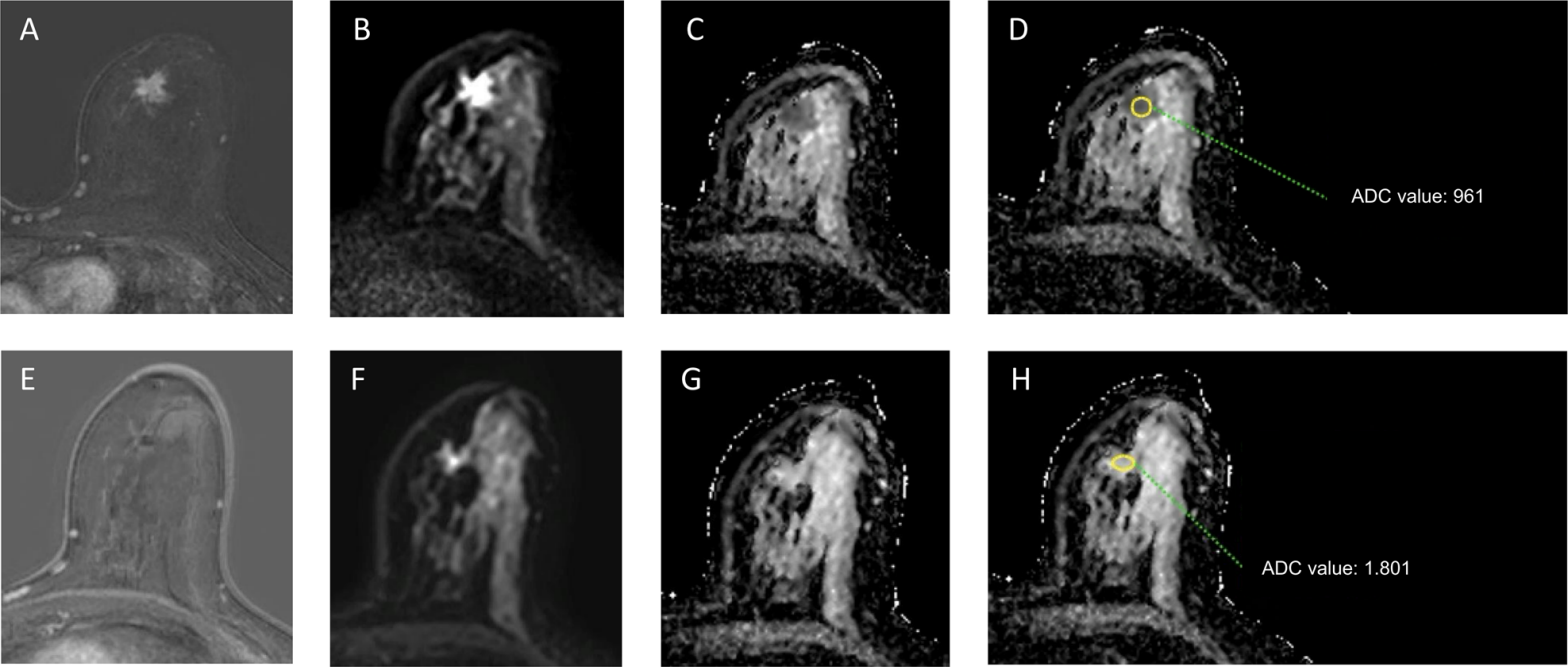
Assessment of ADC value in a patient with radiological complete response at contrast-enhanced MRI breast. A–D show pre-NST images and E–H show the post-NST images. A, E are subtracted images showing the tumor area (the dark spot in E is due to the clip inserted at the tumor site). B, F show the corresponding area at the b800 images. C, G show the corresponding ADC maps. D, H show the ROIs used with the corresponding ADC values in 10^−6^ mm^2^/s
